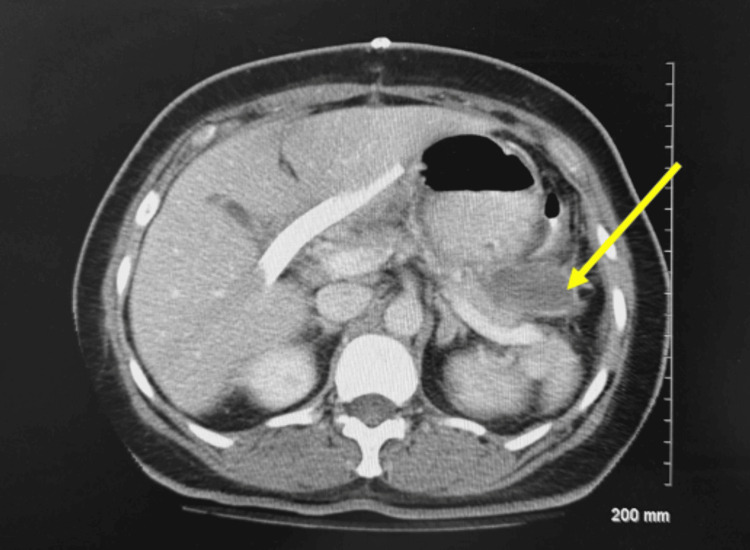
CT Abd/Pelvis with IV contrast on POD 13 showing a fluid collection of 4.7cm in diameter (as represented by the yellow arrow) with an enhancing rim and a small outpouching anteriorly and superiorly that contains a gas bubble. That segment of collection is 14 mm in diameter and consistent with a well-defined abscess.
